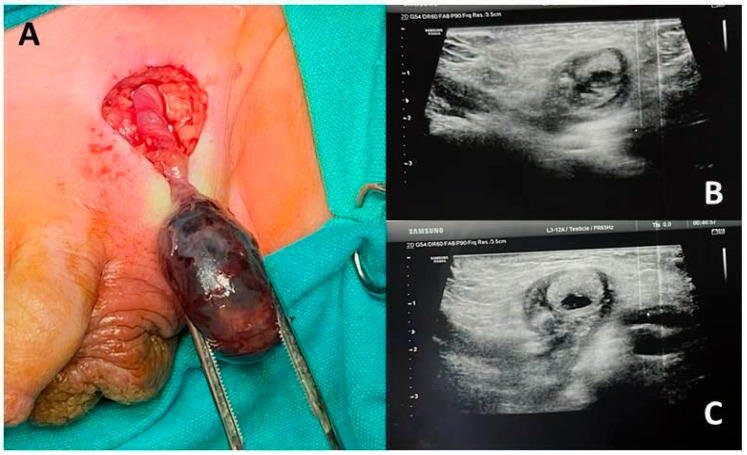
(A) Intraoperative appearance of left necrotic testicle and the twisted spermatic cord. (B,C) Ultrasound appearance of the testicle: the iso/anechoic image reveals an evident anechoic colliquative necrosis area.
